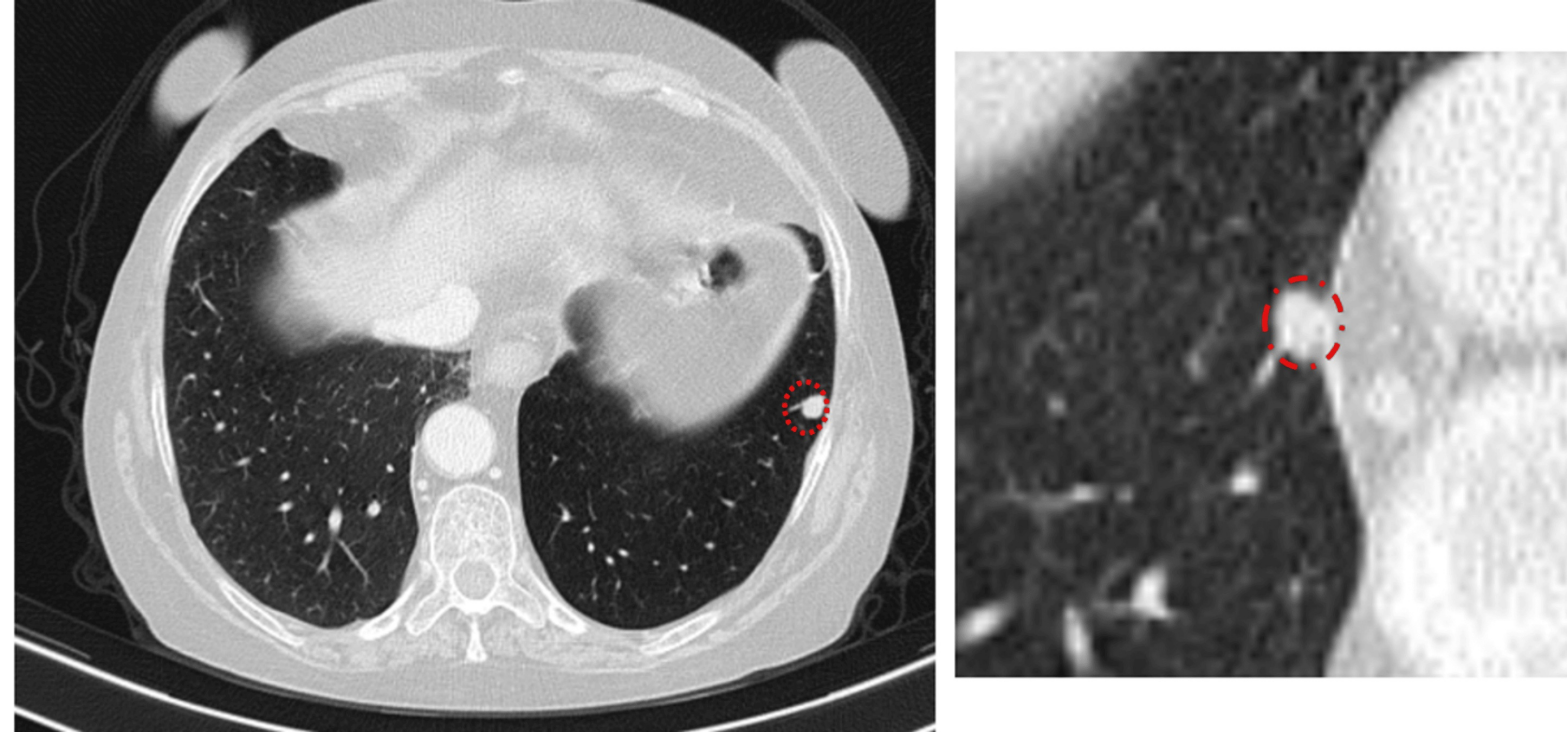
Post-chemotherapy axial and magnified CT images demonstrating a reduction in the number of pulmonary nodules and micronodules, consistent with a partial therapeutic response.
